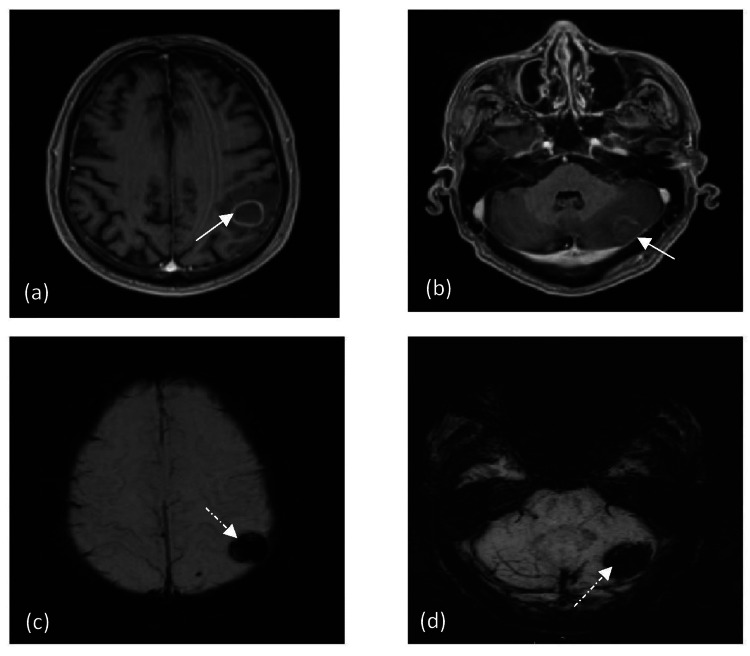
MRI brain T1WI axial contrast MRI (a and b) images depicting supra and infratentorial brain metastases with peripheral contrast enhancement and surrounding oedema (white solid arrows). Susceptibility weight imaging (SWI) (c and d) demonstrating corresponding blooming artefact (white dashed arrows) keeping with hemosiderin deposition.
